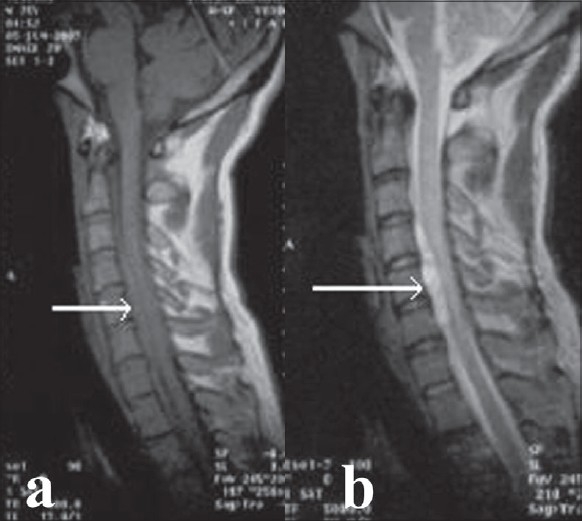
Mid sagittal T1 (a) and T2 (b) weighted MRI of cervical spine at onset of weakness shows epidural mass (solid arrow)
